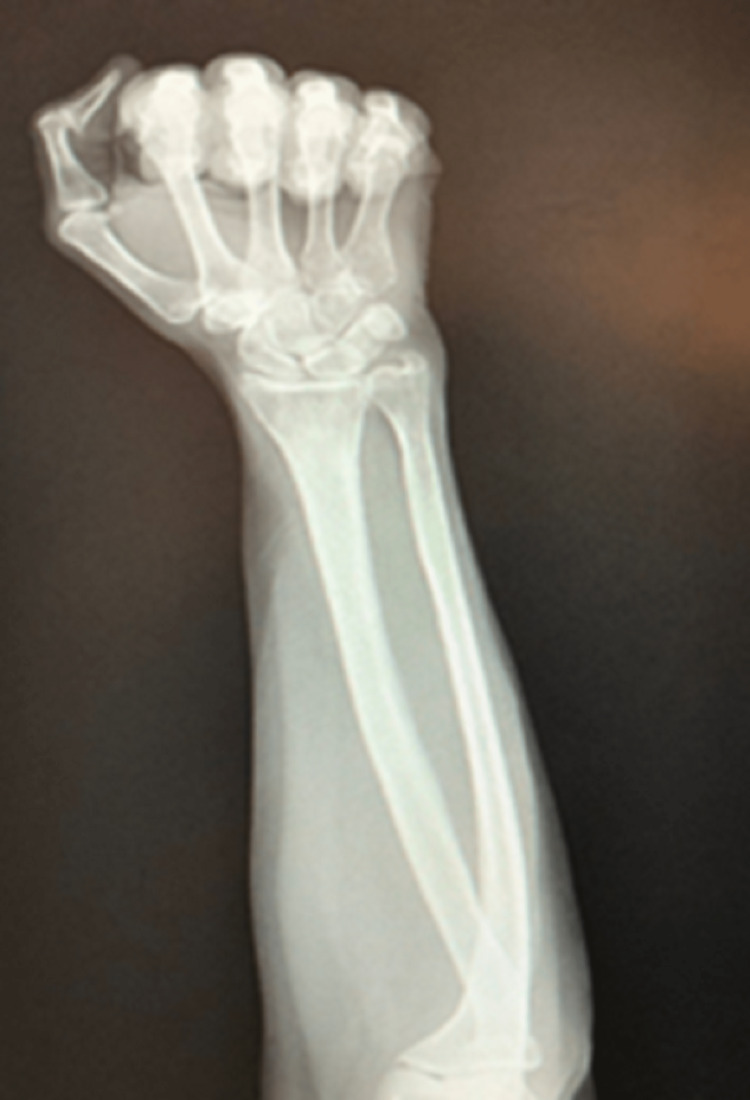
Left forearm radiograph - anterior-posterior view Radiograph of the patient’s left elbow and wrist from an anterior-posterior (AP) view. This radiograph does not show any immediate structural defects of the patient’s left radius, ulnar, carpal, and phalangeal bones. Other findings which are present include a small calcification within the left triangular fibrocartilage complex area near the left ulnar styloid process as well as a possible bone contusion about the left lateral epicondyle.
